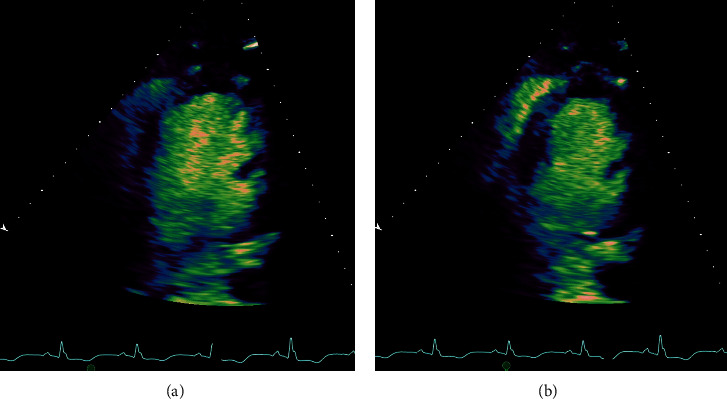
Transthoracic echocardiogram on admission demonstrating severe left ventricular (LV) systolic dysfunction with an ejection fraction of 20%. (a) Two-chamber view of LV end diastole and (b) LV end systole.
